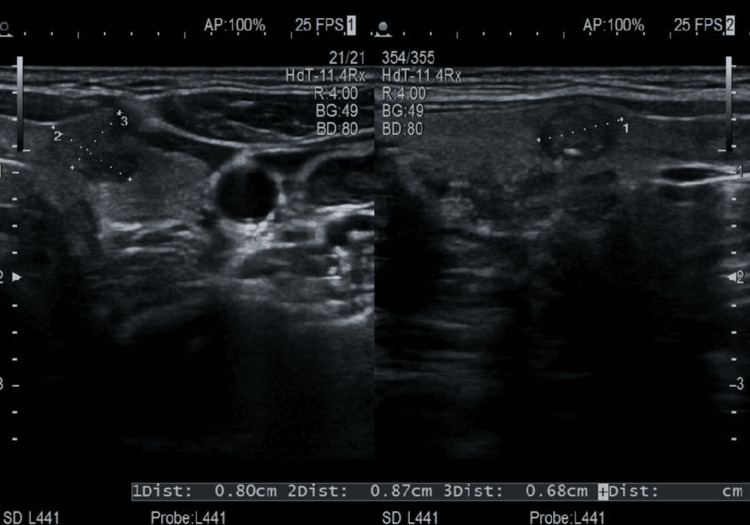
Transverse thyroid ultrasound Transverse B-mode ultrasound image showing multiple hypoechoic nodules in the left thyroid lobe, the largest measuring approximately 0.87 × 0.80 × 0.68 cm.
